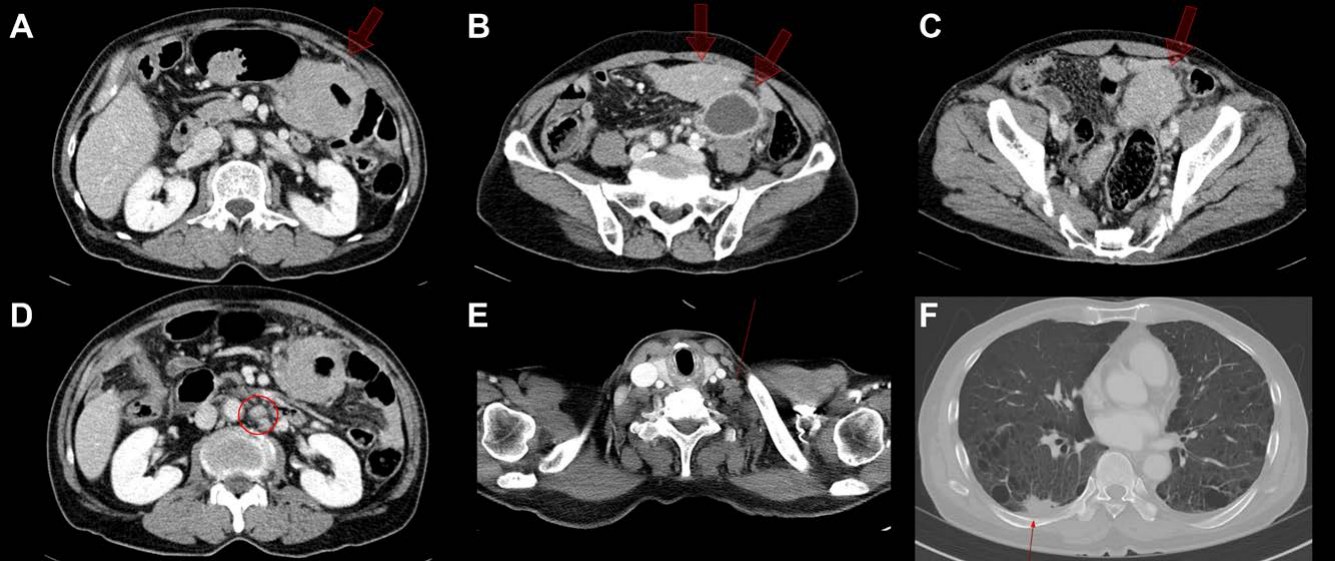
Computed tomography scan showing 3 masses with soft tissue density encasing the transverse and sigmoid colon (white arrows in A–C) and enlarged lymph nodes at the mesentery (white arrow in D) and supraclavicular fossa (blue arrow in E). A pleural mass (white arrow in F) and a 4.5 cm sized intra-abdominal abscess (blue arrow in B) are also seen.
